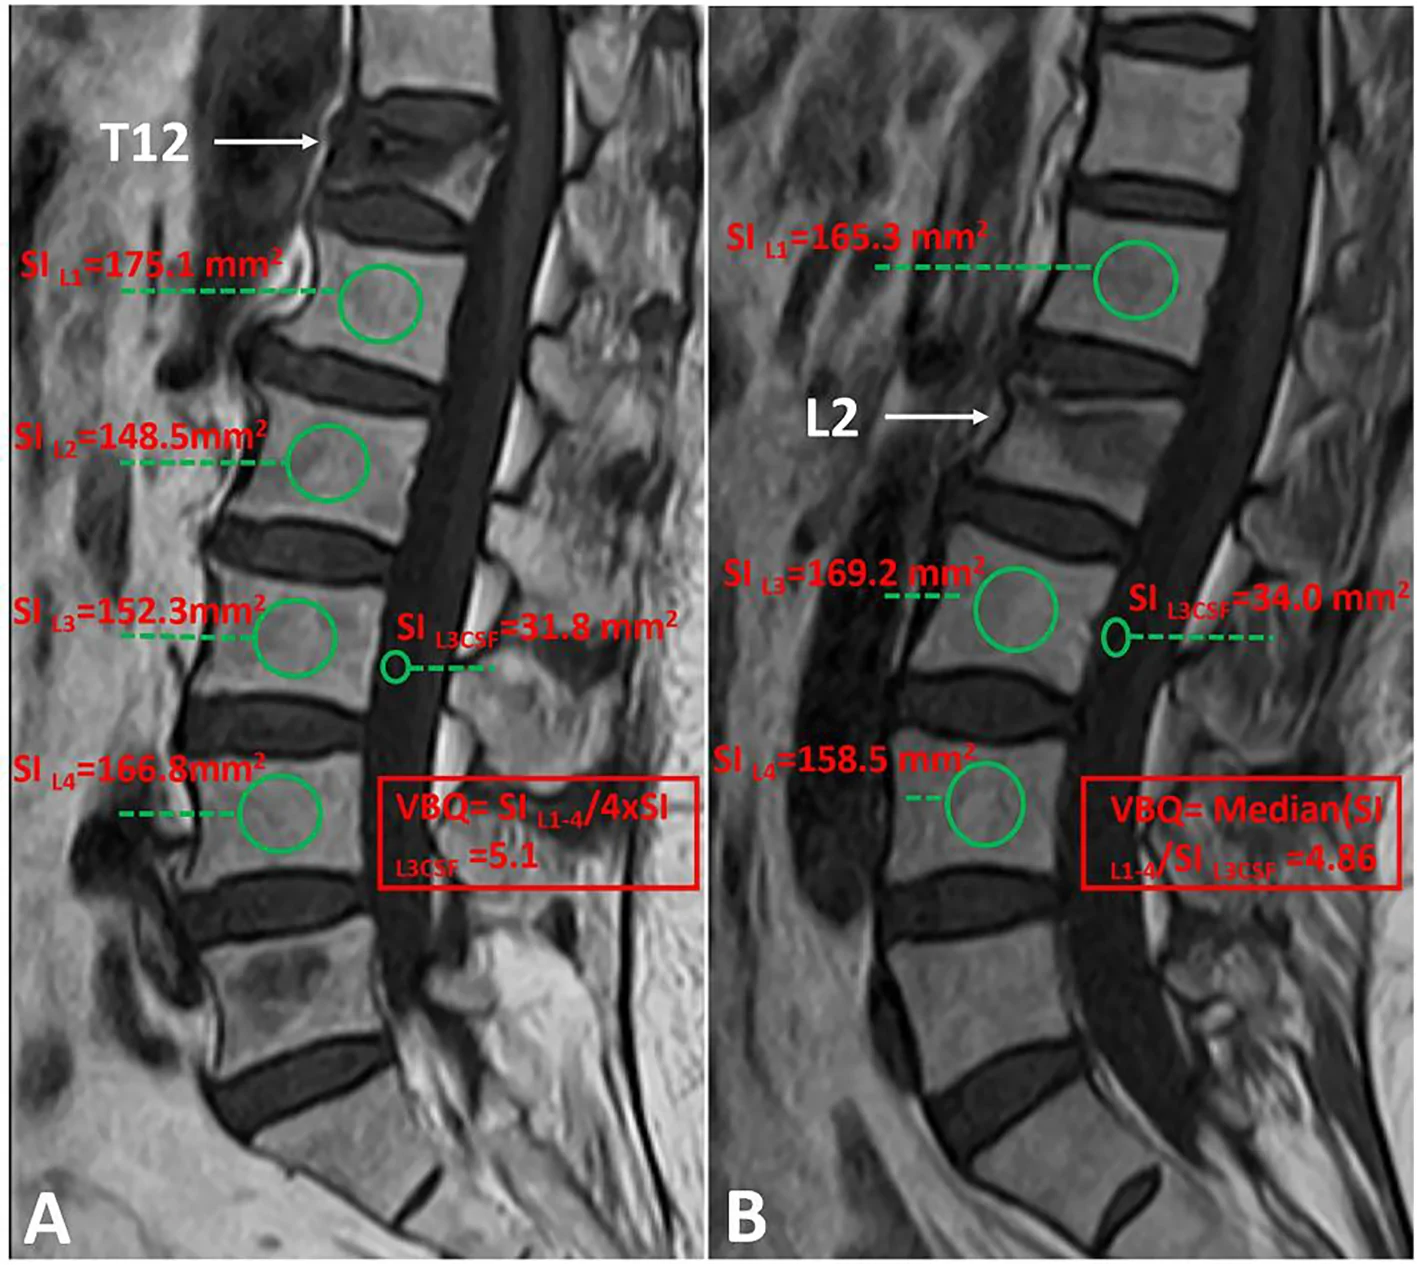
VBQ score measurement on non-contrast T1-weighted MRI. (A) For fractures outside the L1-L4 segment, the VBQ score was calculated as the mean SI of L1-L4 divided by the SI of CSF at L3. (B) For fractures within the L1-L4 segment, the VBQ score was determined by the median SI of L1-L4 divided by the SI of CSF at L3.
